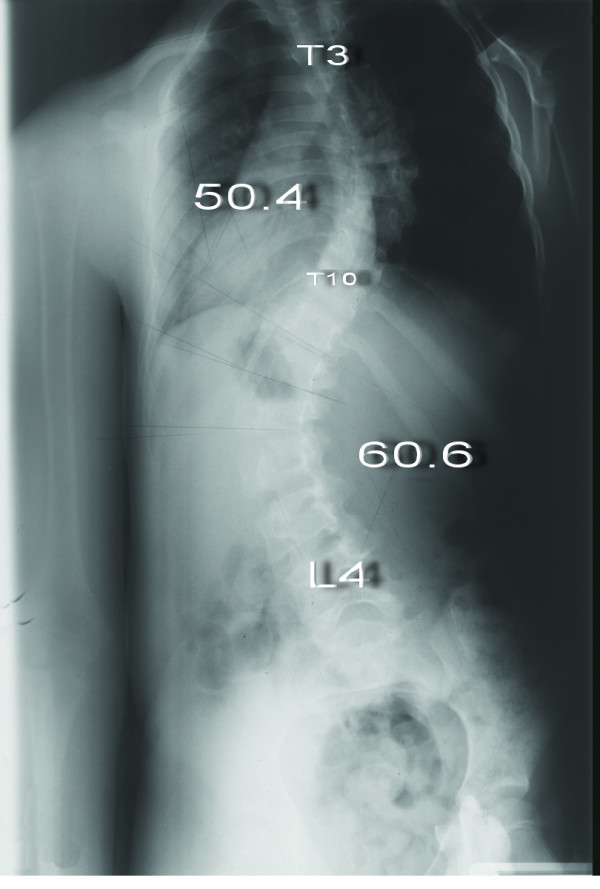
Eight-year-old girl with Lenke type 3CN scoliosis (Patient no 7) Preoperative anteroposterior radiograph shows 50.4° thoracic curve and 60.6° lumbar curve.
